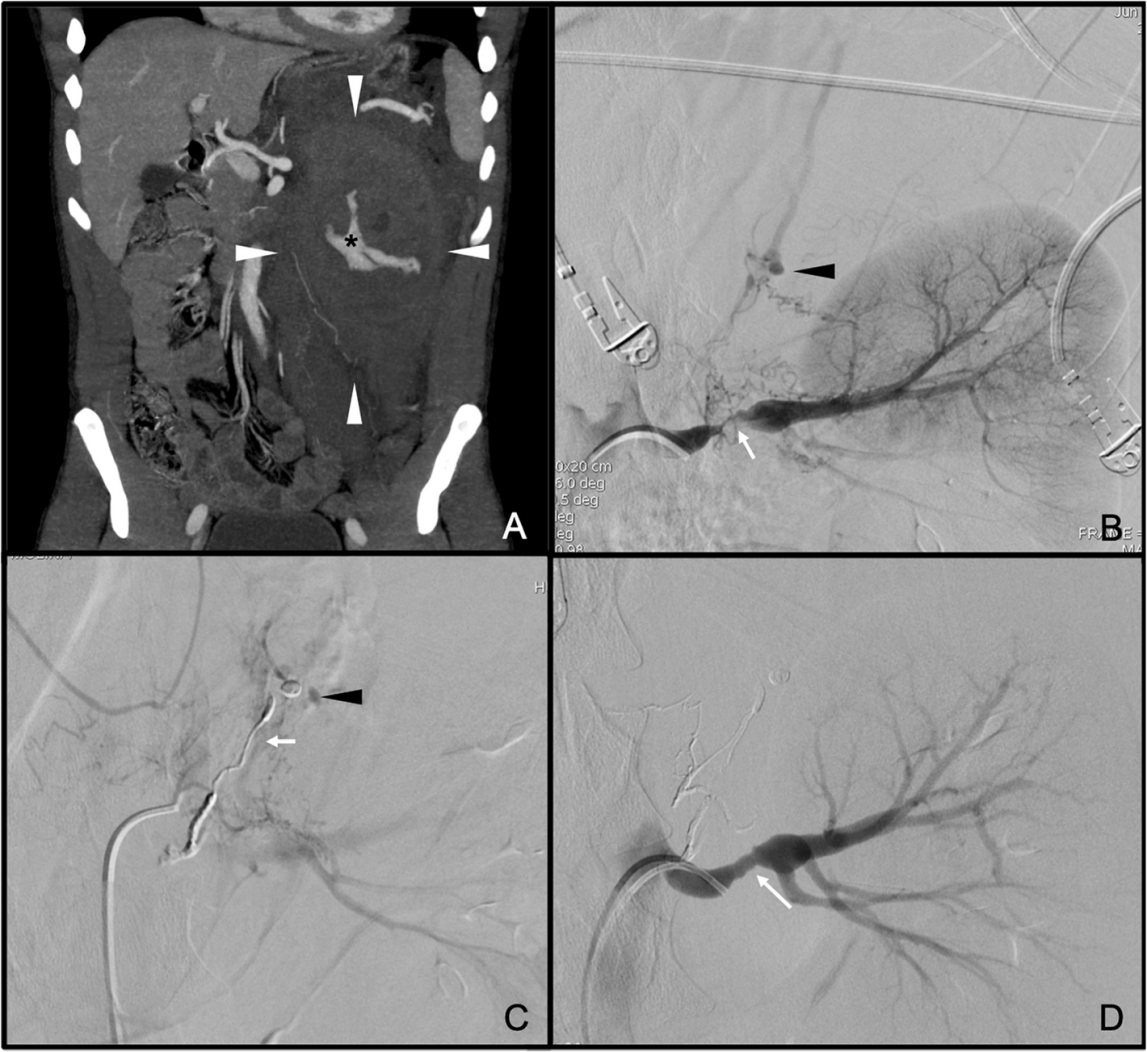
a Angio-CT, coronal plane. Left retroperitoneal haematoma (white arrowheads), with active bleeding inside (black asterisk). b Selective arteriography of the left renal artery, with contrast extravasation (black arrowhead) dependent on vascular network, and stenosis of the renal artery (white arrow). c Embolisation material in the vascular network (white arrow), new contrast extravasation point dependent on adrenal aortic branch (black arrowhead). d Control after embolisation and angioplasty, without identifying contrast extravasation points and with partial recovery of the stenosis (white arrow)
